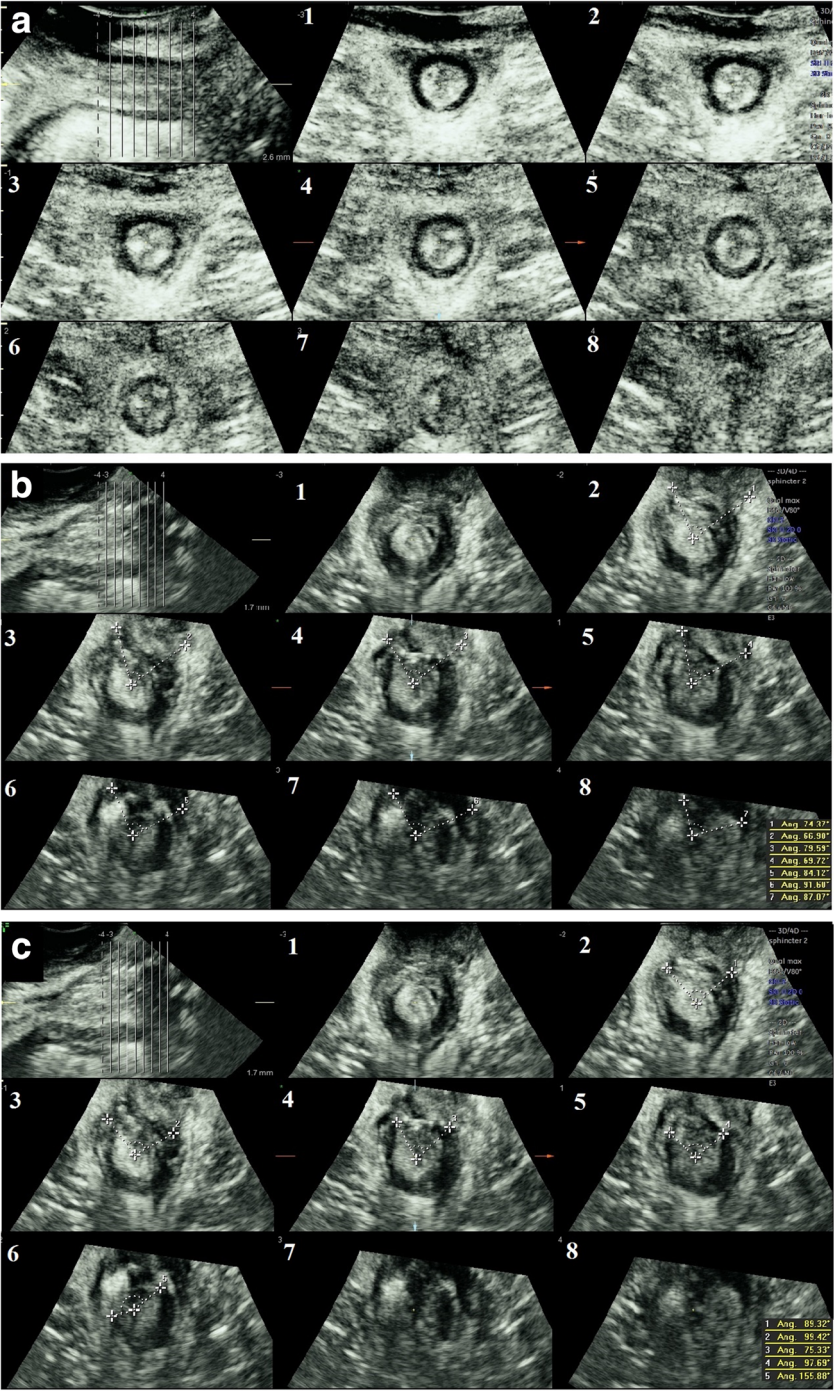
Introital/transperineal ultrasound of the anal sphincters. Tomographic ultrasound imaging demonstrating the puborectalis level (*slice 1*), the deep level (*slice 2*) of the internal and external anal sphincters, the superficial level (*slices 3–6*) of the internal and external anal sphincters and the subcutaneous level (*slices 7–8*) of the external anal sphincter. a Intact sphincters. b Defect in the external anal sphincter indicated with *dotted lines*. c Defect in the internal anal sphincter indicated with *dotted lines*
